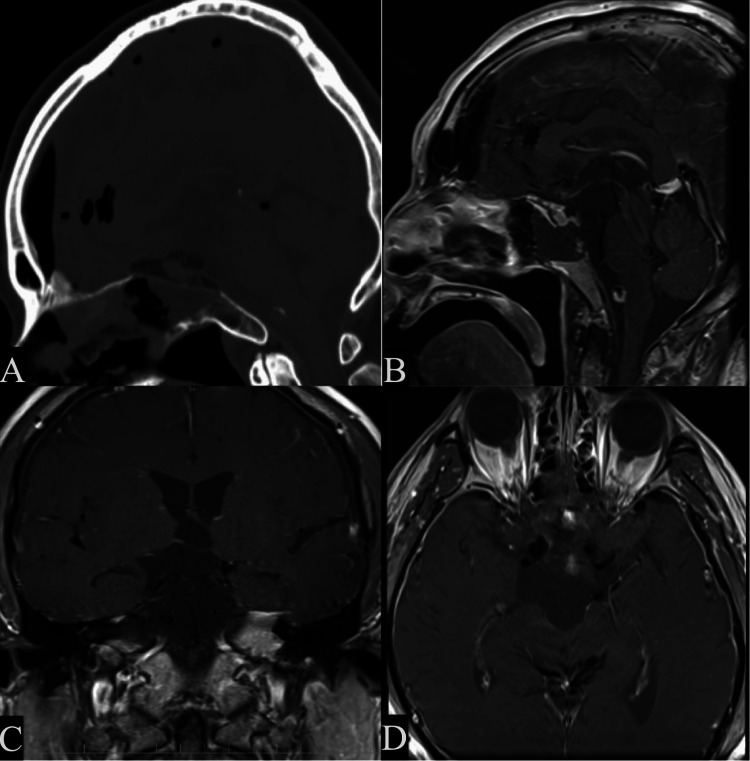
Postoperative CT and MRI images (A) A sagittal CT image demonstrates adequate bone removal from the planum sphenoidale, sellar floor, and dorsum sellae in the endoscopic endonasal transplanum and transdorsum sellar approach. (B-D) Postoperative contrast-enhanced MRI demonstrating total resection of the craniopharyngioma
